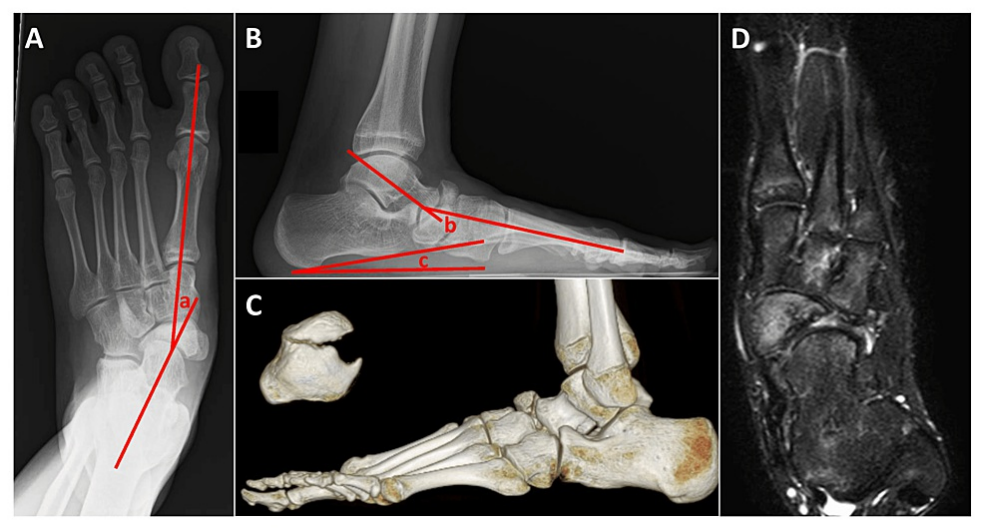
Imaging of the bipartite navicular (A) Anteroposterior view of the weight-bearing radiogram; (B) Lateral view of the weight-bearing radiogram; (C) Computed tomography (CT); (D) T2-weighted fat-suppressed magnetic resonance imaging. a, b: talo-first metatarsal angle; c: calcaneal pitch
